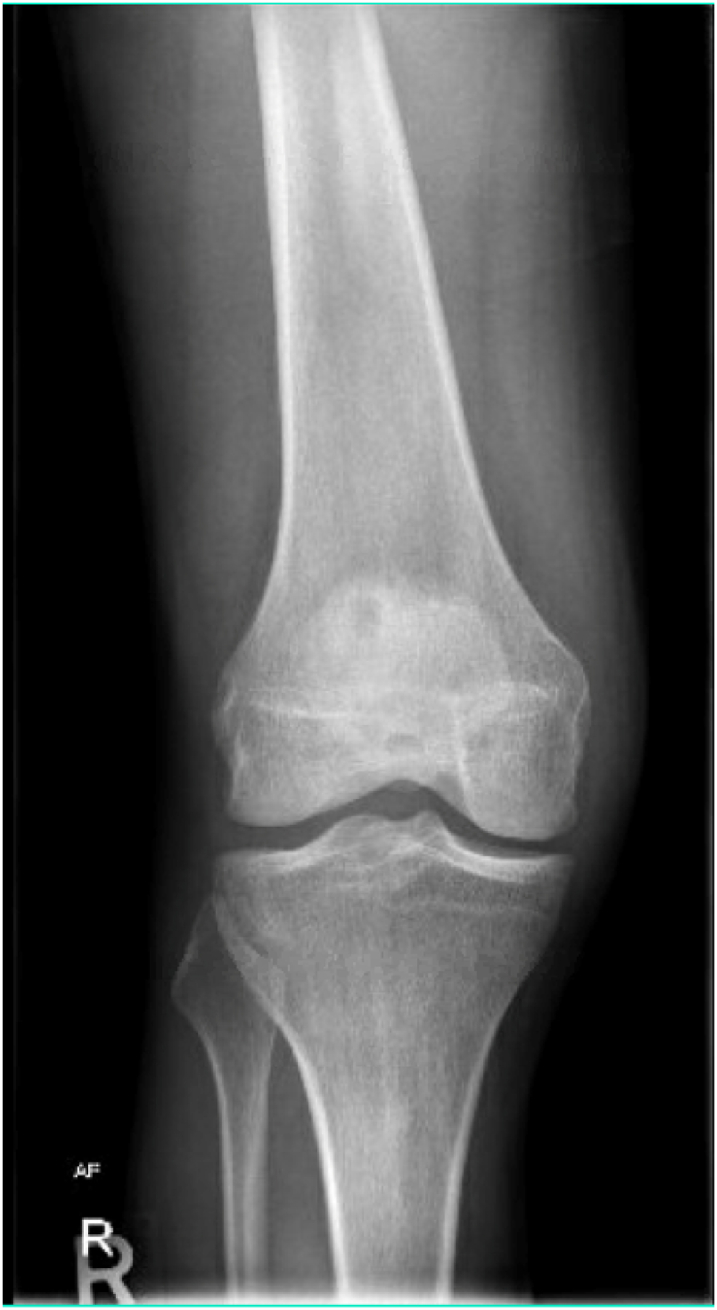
Right knee AP view normal x-ray.
